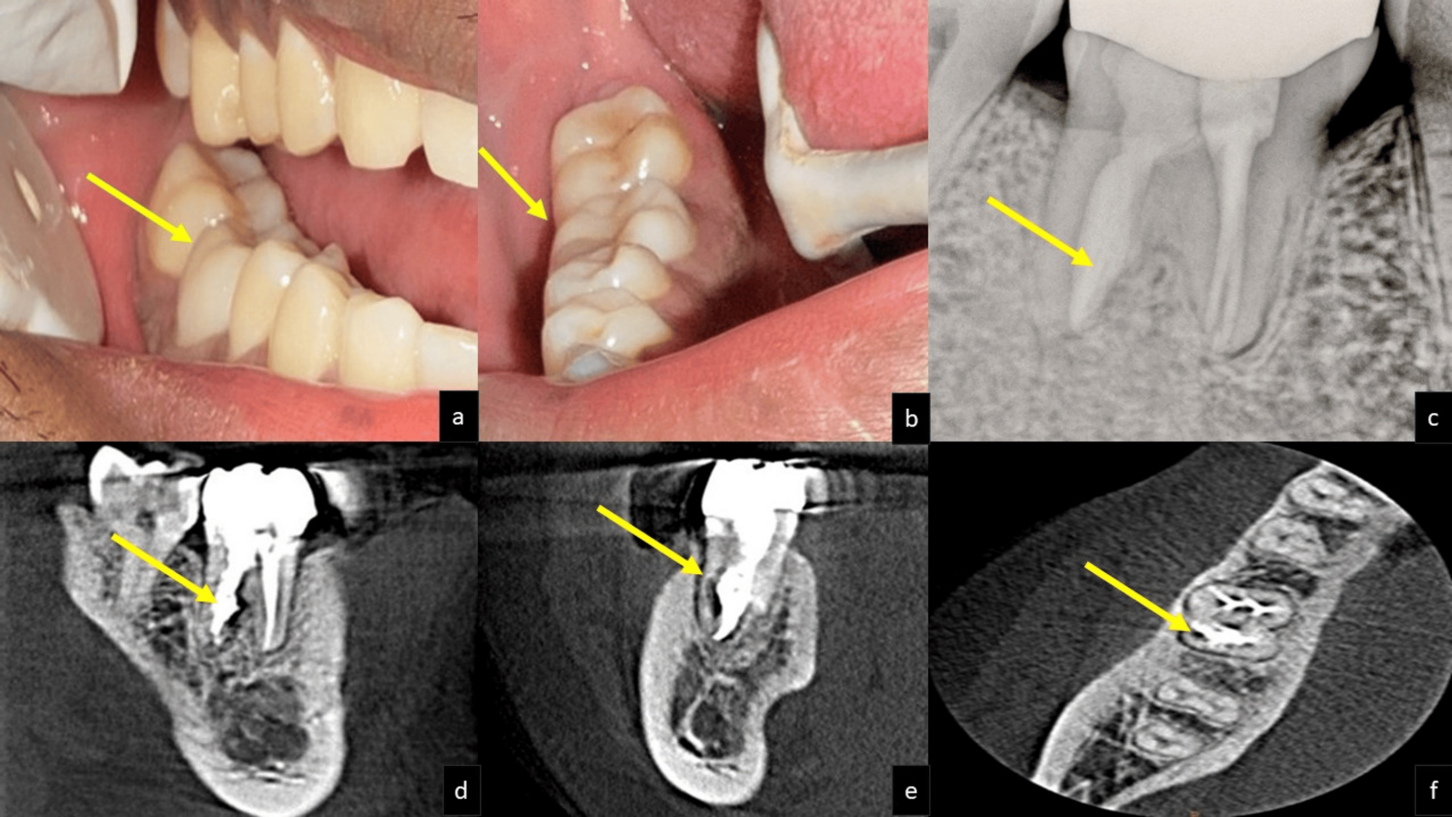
On a 12-month review. (a) Intraoral buccal view, (b) intraoral lingual view, (c) intraoral periapical radiograph (RVG), (d) CBCT-sagittal view, (e) coronal view, and (f) axial view. CBCT: cone beam computed tomography; RVG: radiovisiography.
